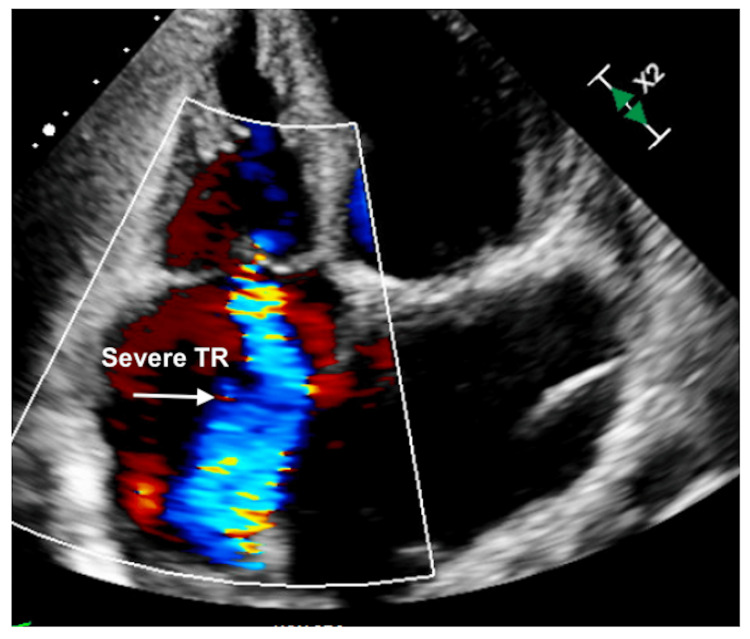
Transthoracic echocardiography four-chamber view showing severe TR TR: Tricuspid regurgitation
